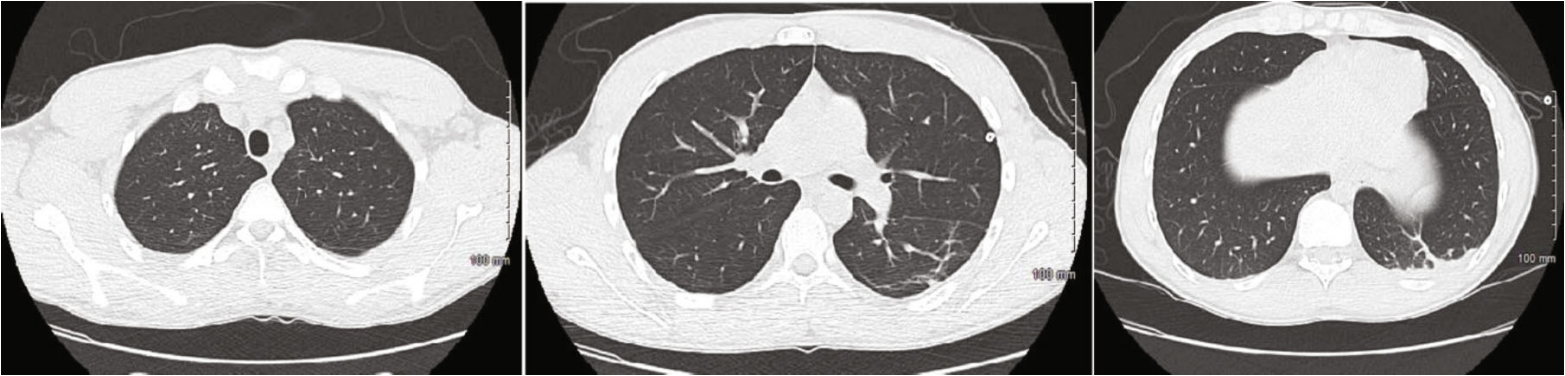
Chest CT showing left-sided atelectasis.
